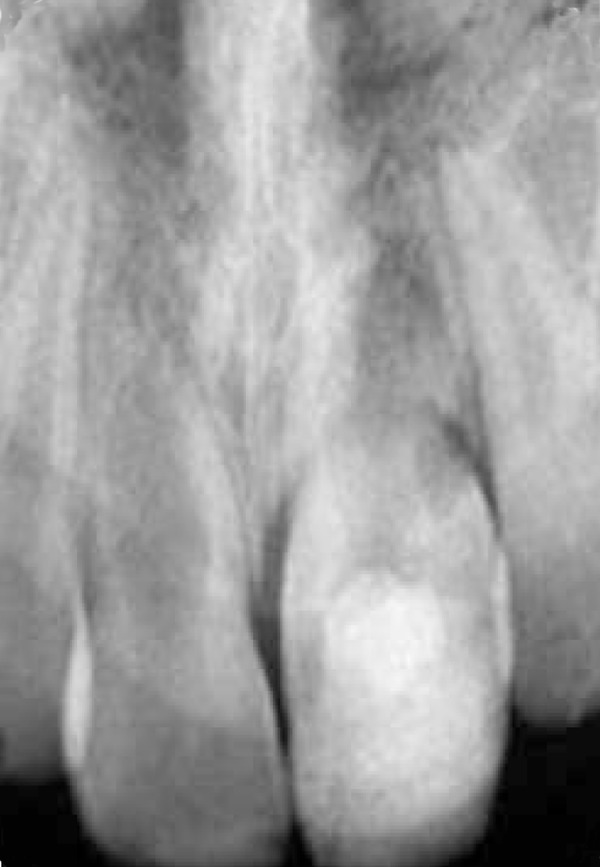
Radiograph taken 5 years after avulsion
